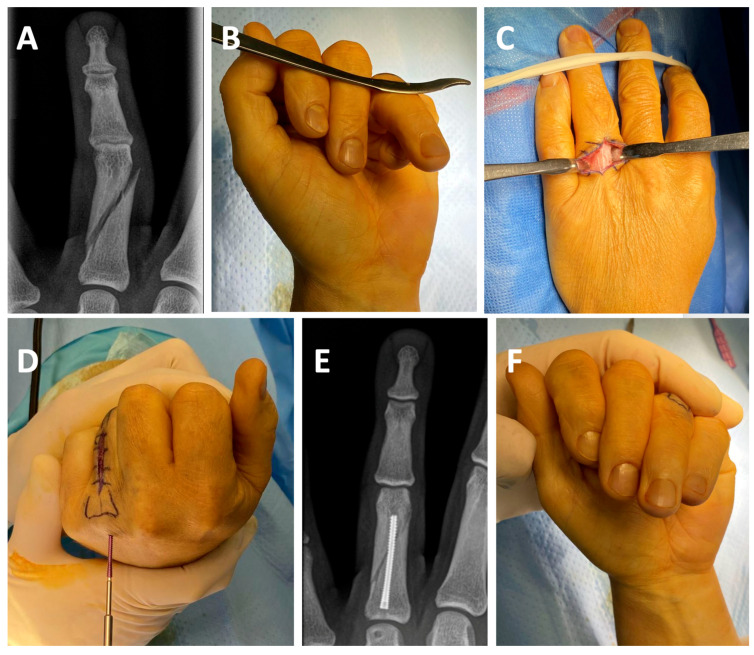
Spiral extra-articular fracture of the proximal phalanx. (A). Preoperative anteroposterior radiograph showing a displaced fracture. (B). Intraoperative clinical image that demonstrates marked clinodactyly due to malalignment. (C). Mini-open dorsal approach through a longitudinal skin incision and midline split of the extensor tendon, enabling direct visualization and accurate fracture reduction. (D). Transarticular insertion of a fully threaded intramedullary headless compression screw. (E). Postoperative radiograph confirming anatomic fracture reduction and proper positioning of a stabilizing K-wire. (F). Intraoperative clinical image after the procedure, demonstrating corrected alignment.
